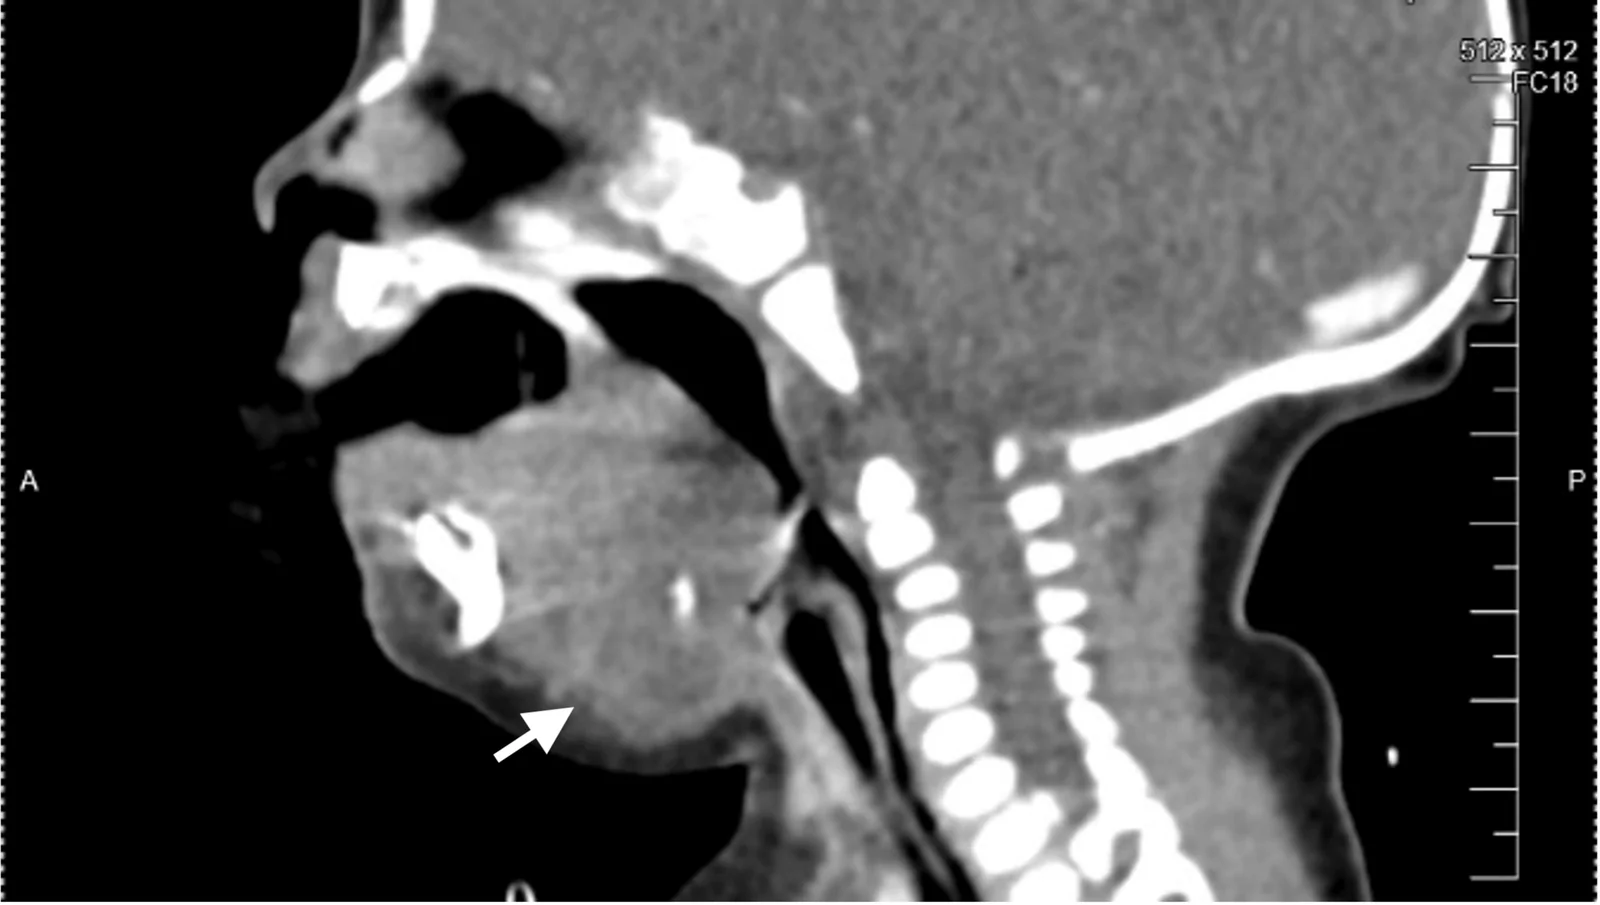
Sagittal computed tomography (CT) image of the vallecular cyst (arrow) before drainage.
